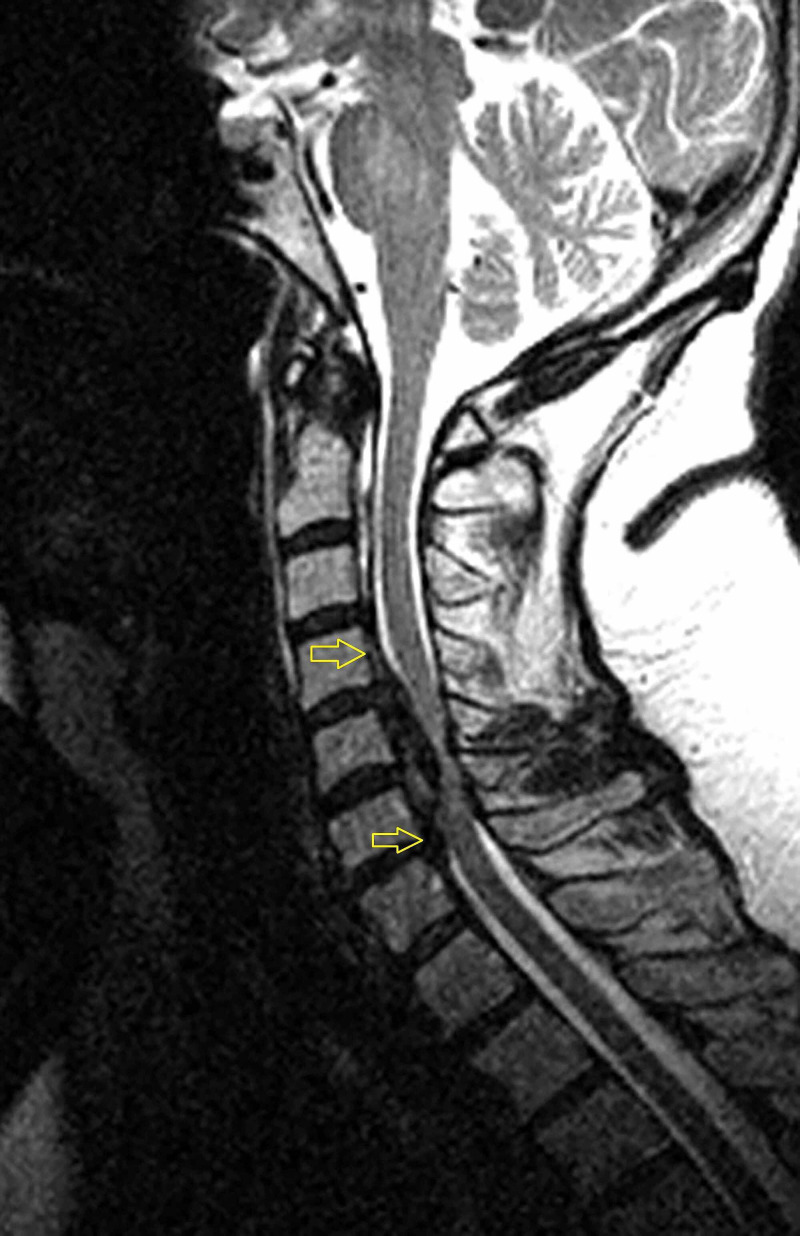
Sagittal T2 sequence magnetic resonance imaging scan showing severe spinal cord impingement by anterior compressive disease spanning C4 to C6 (yellow arrows).
